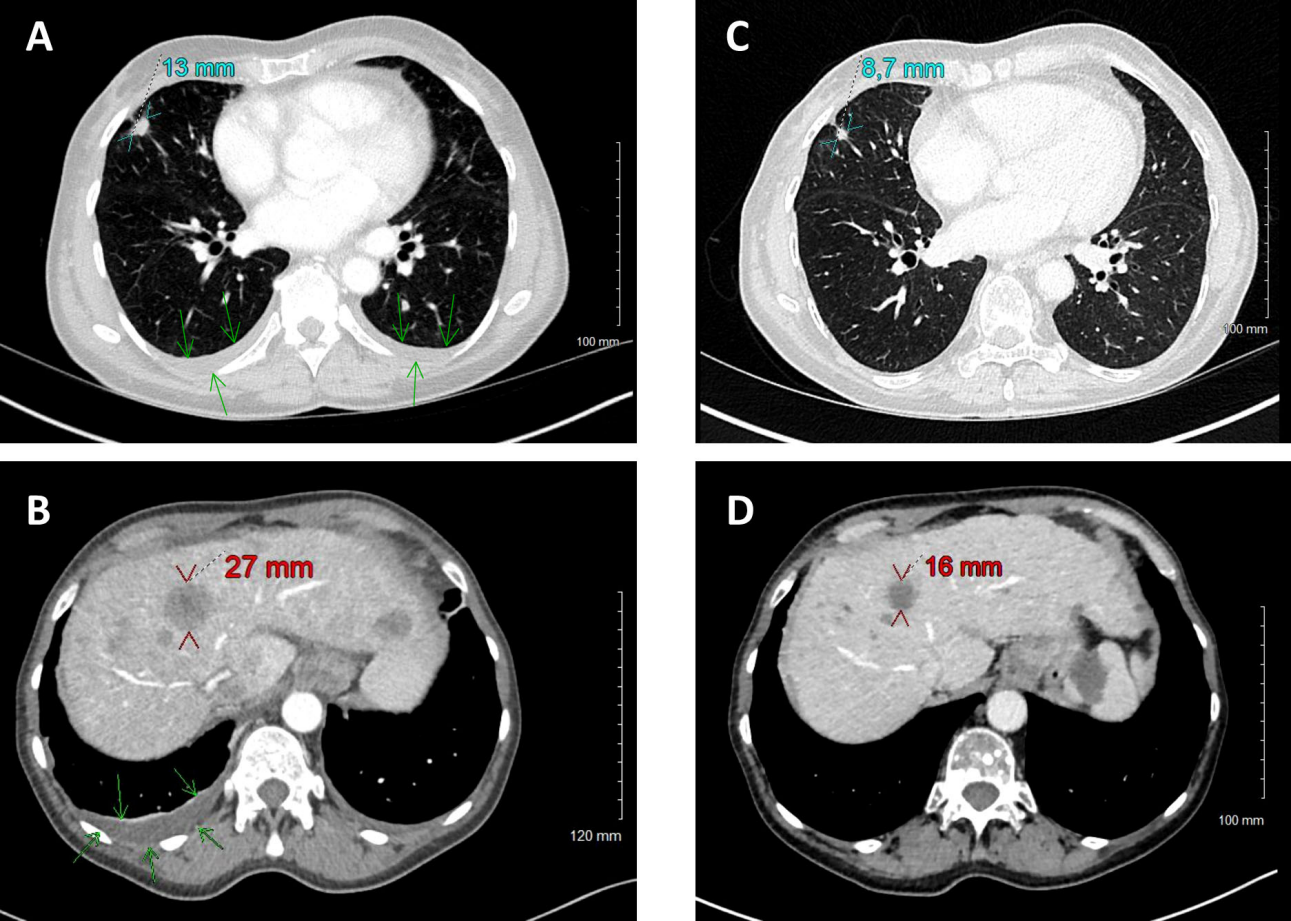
Comparison of CT scans before (June 7, A, B) and with osimertinib treatment (August 29, C, D). Size reduction of primary tumor (cyan) and target hepatic metastasis (red). Disparition of pleural effusion (green arrows).
